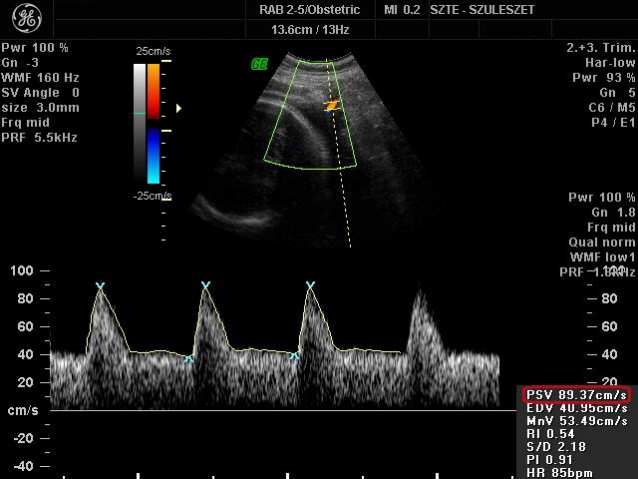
Measurement of uterine artery peak systolic velocity (AUtPSV) taken from the sagittal section of the uterus with color flow mapping and applied pulsed wave Doppler. PSV value is highlighted in red.
